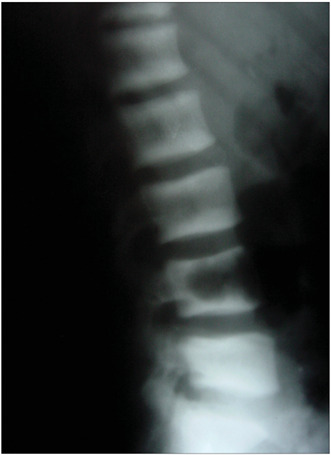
Lumbar column radiograph (lateral view) showing band-like regions of increased opacity (osteosclerosis) at the upper and lower margins, with normal density in the middle parts of the vertebral bodies, which is typical of the “rugger jersey spine” sign.
